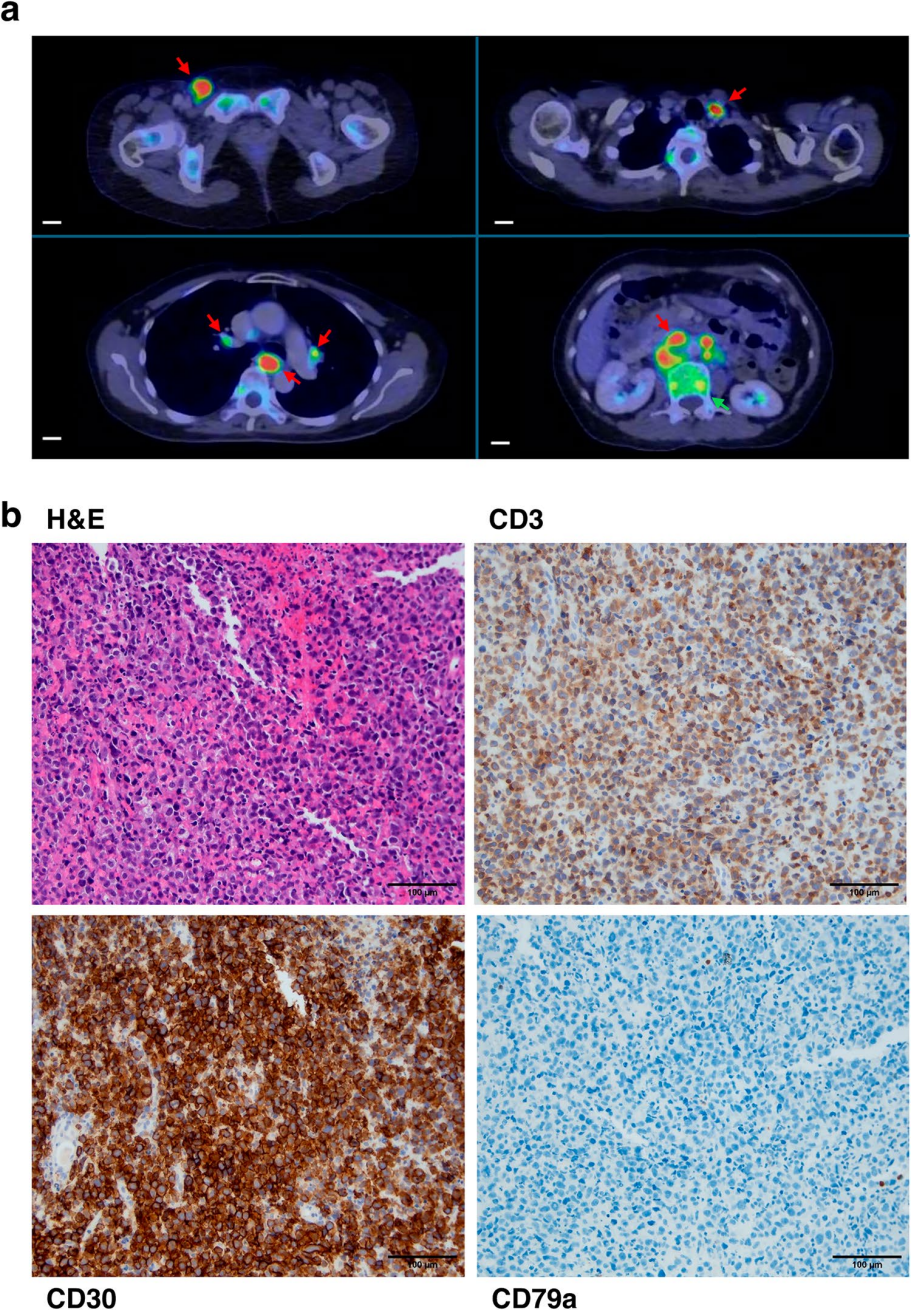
Clinical and pathological features of patient with ALK-negative ALCL. a 18F-fluorodeoxyglucose Positron Emission Tomography/Computed Tomography (FDG-PET/CT) imaging taken at time of relapse showed hypermetabolic supradiaphragmatic and infradiaphragmatic lymphadenopathy (red arrows), including the right inguinal, left supraclavicular, subcarinal, peri-hilar and para-aortic regions, as well as FDG-avid bony lesions indicative of marrow infiltration (green arrow) (scale bar: 2 cm). Transverse diameter of dominant nodes: (Top Left to Right) 2–1.2 cm (Bottom Left to Right) 1.7–5 cm. b IHC of patient tumour tissue revealed to be strongly and uniformly positive for CD30 and CD3, while being negative for CD79a (scale bar: 100 µm).
